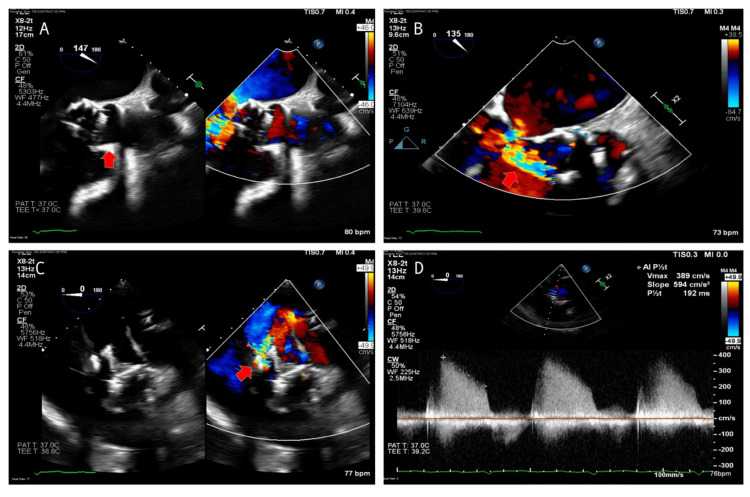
TEE of the prosthetic aortic valve structure. (A) Mid-esophageal (ME) long axis view (red arrow-anterior aortic valve annulus) showing valve structure dehisced from the anterior annulus. (B) ME long axis view zoomed (red arrow-paravalvular regurgitant flow) showing paravalvular regurgitant jet. (C) Deep transgastric (DTG) view (red arrow-paravalvular regurgitant flow) showing valvular structure dehiscence and paravalvular regurgitant jet. (D) Continuous wave Doppler measurement (pressure half-time 192 ms) consistent with severe aortic regurgitation. TEE: transesophageal echocardiogram.
